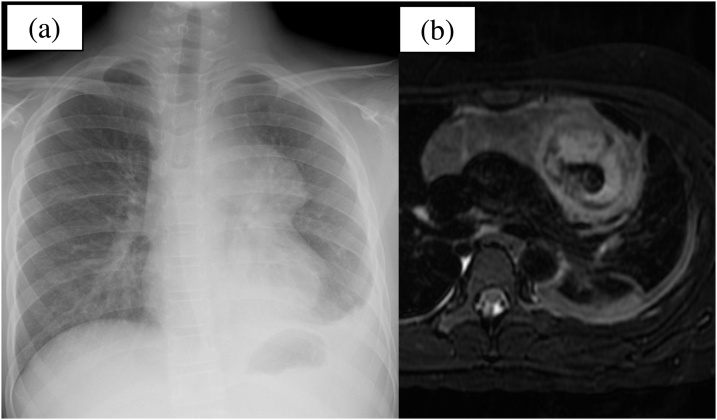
Patient images on the second day of admission. (a) Chest radiography showing that the tumour’s shadow has increased and the left costal phrenic angle is dull. (b) Magnetic resonance imaging revealing pleural effusion and intratumoral haemorrhage.
